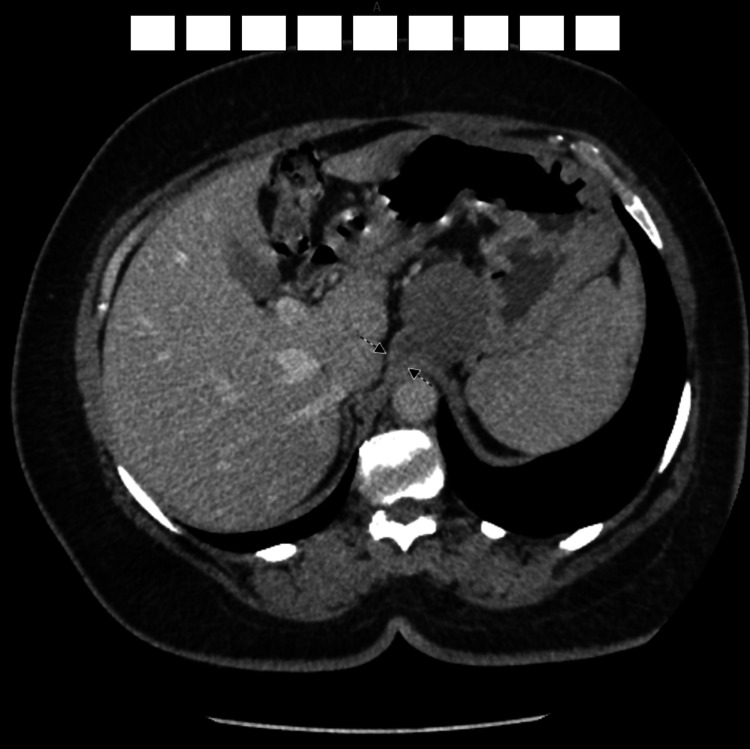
CT scan The aforementioned findings are suggestive of a foregut duplication cyst, such as a bronchogenic cyst arising from the diaphragmatic crus. A pseudocyst is another less likely possibility in the presence of a history of prior pancreatitis Black arrows pointing to the possible origin of the cyst (right crus) CT: computed tomography
